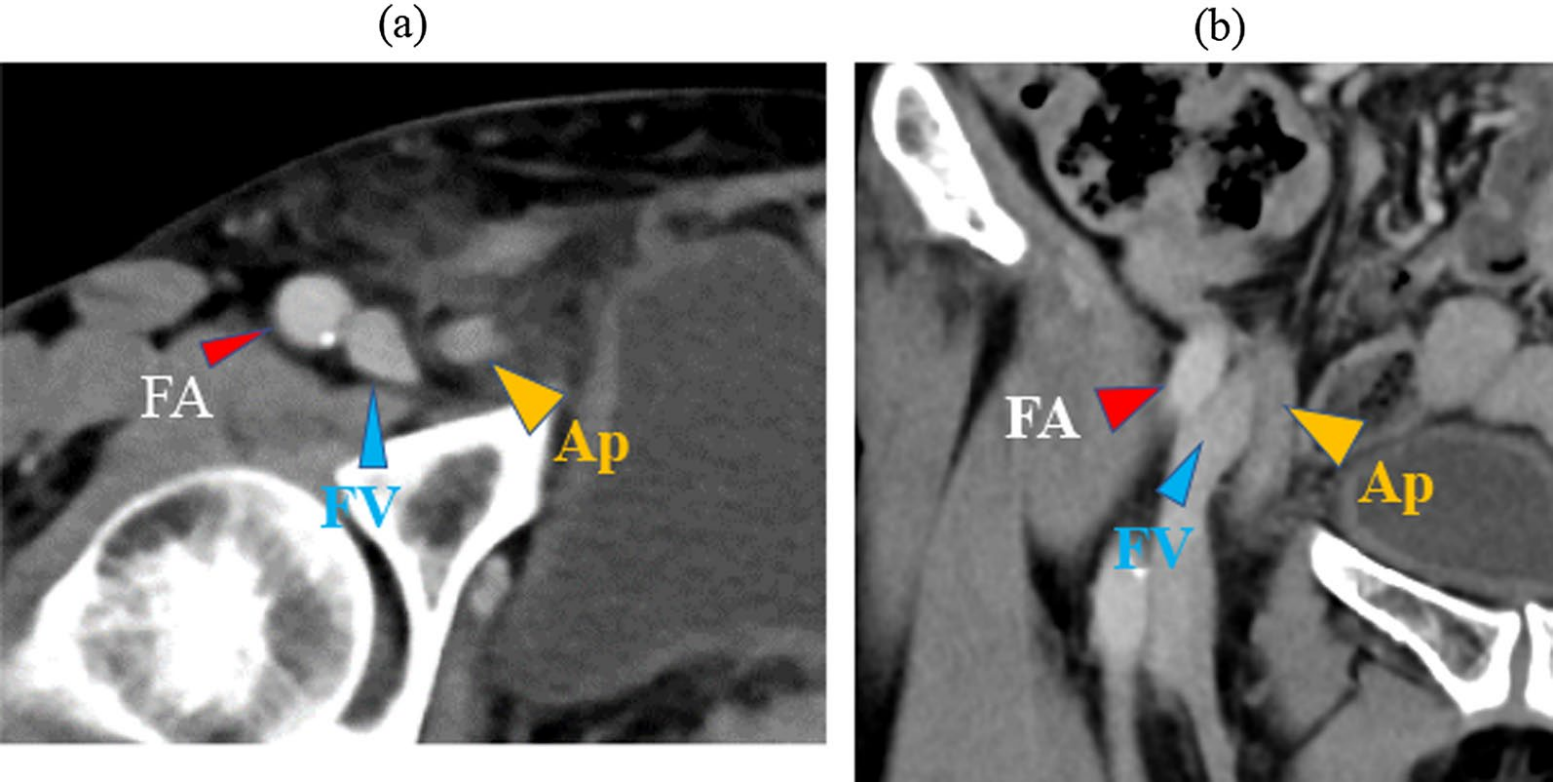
Computed tomography (Case 2). a Axial image showing a well-defined structure 6 mm in diameter (Ap) with contrast enhancement on the medial side of the right femoral vein (FV). b Coronal image showing a tubular structure continuous with the cecum. *Ap* appendix, *FA* femoral artery
